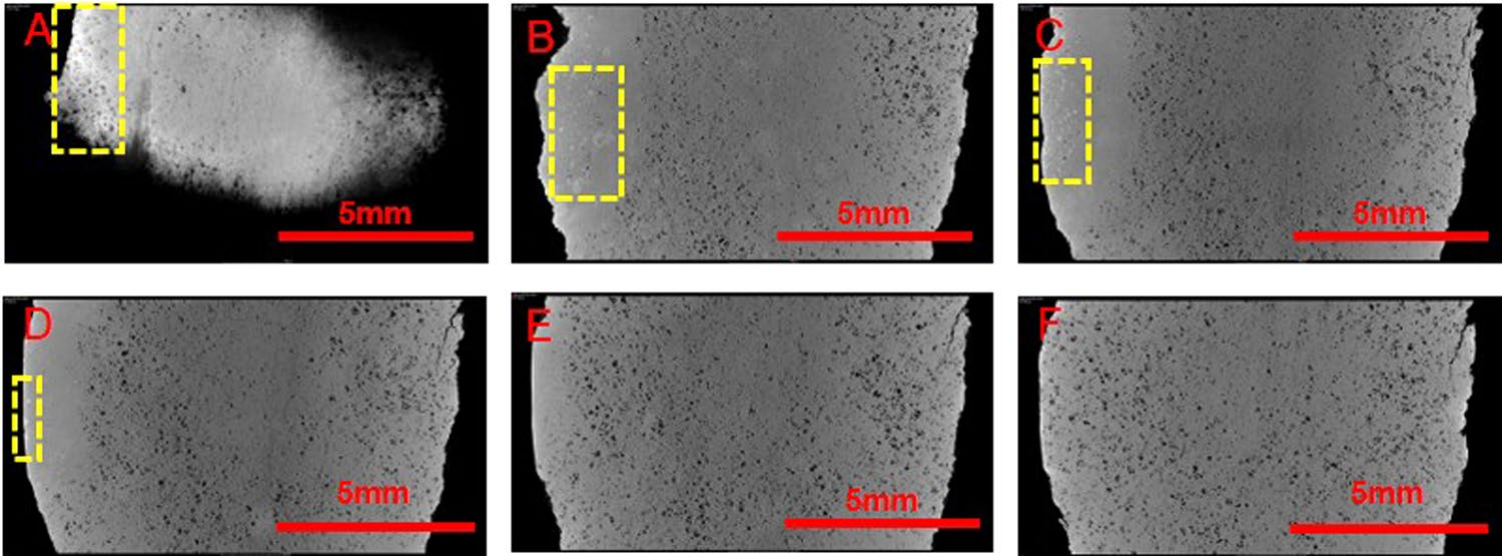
The CT images of G212-1. (A–F) Images are from one side to the other side in turn and the interval of each image is 250 μm. The light spots in the yellow imaginary area are mercury alteration.
